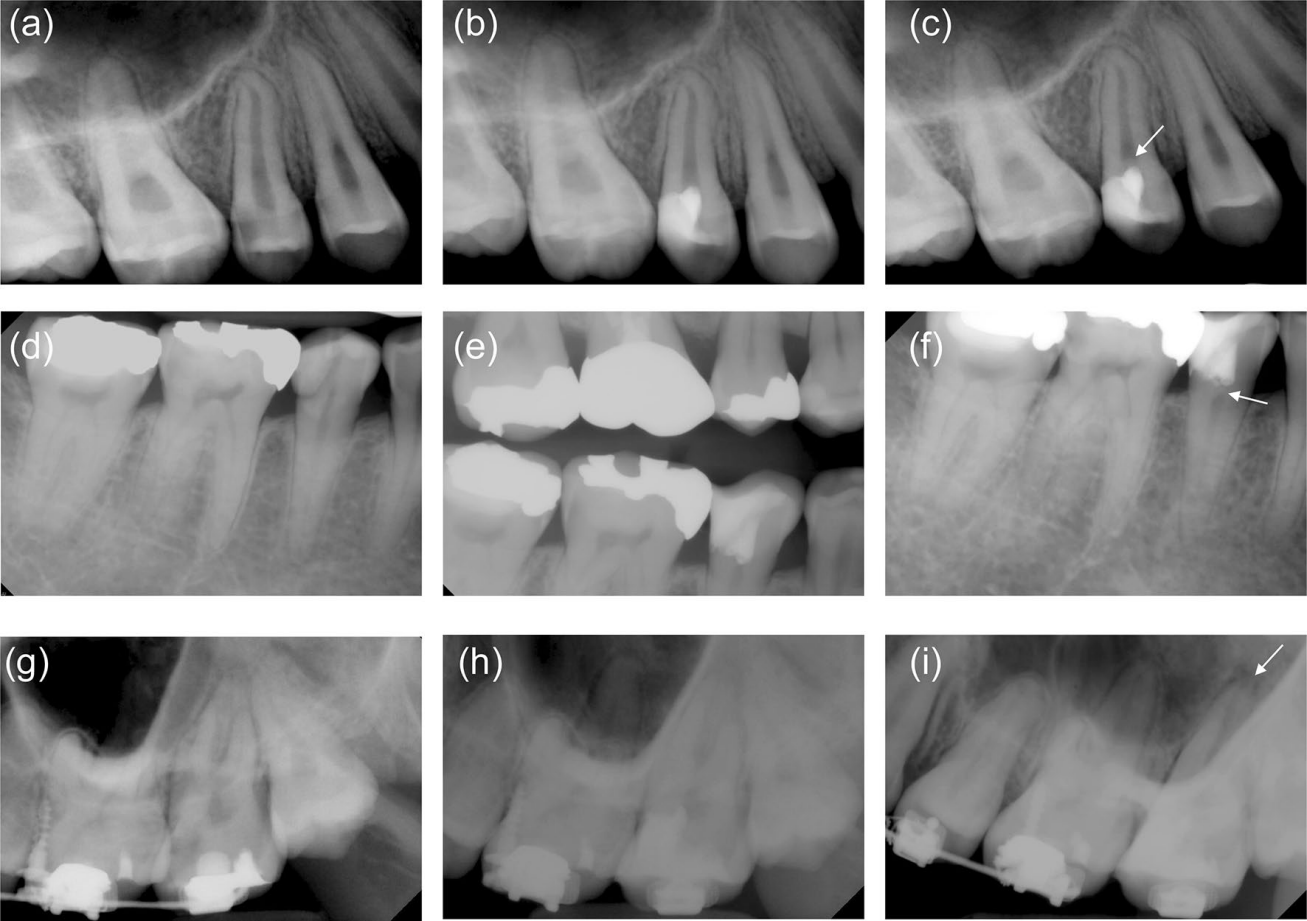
Outcome of teeth treated with partial pulpotomy. (a) Preoperative radiograph of tooth 15 with extensive caries. (b) Immediately postoperative radiographs after partial pulpotomy using Endocem MTA Premixed. (c) 12-month postoperative radiographs showing calcific bridge formation (white arrow) and normal periapical tissue. (d) Preoperative radiograph of tooth 45 showing caries close to the pulp. (e) Immediately postoperative radiographs after partial pulpotomy with ProRoot MTA. (f) 12-month postoperative radiograph showing calcific bridge formation (white arrow) and normal periapical tissue. (g) Preoperative radiograph of tooth 27 with extensive caries. (h) Immediately postoperative radiographs after partial pulpotomy with ProRoot MTA. (i) 2-month postoperative radiograph showing the periapical lesion around the palatal root. The patient complained of severe pain upon cold stimulus.
